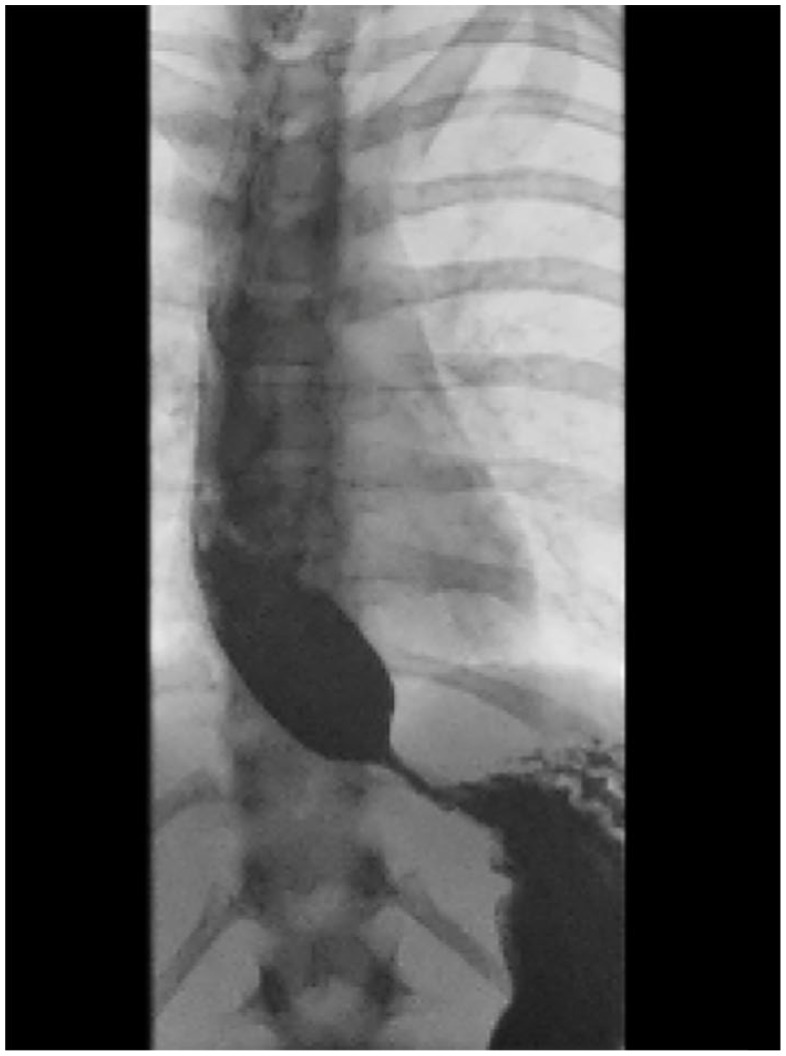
Chest x-ray, barium swallow study showing bird beak sign.
